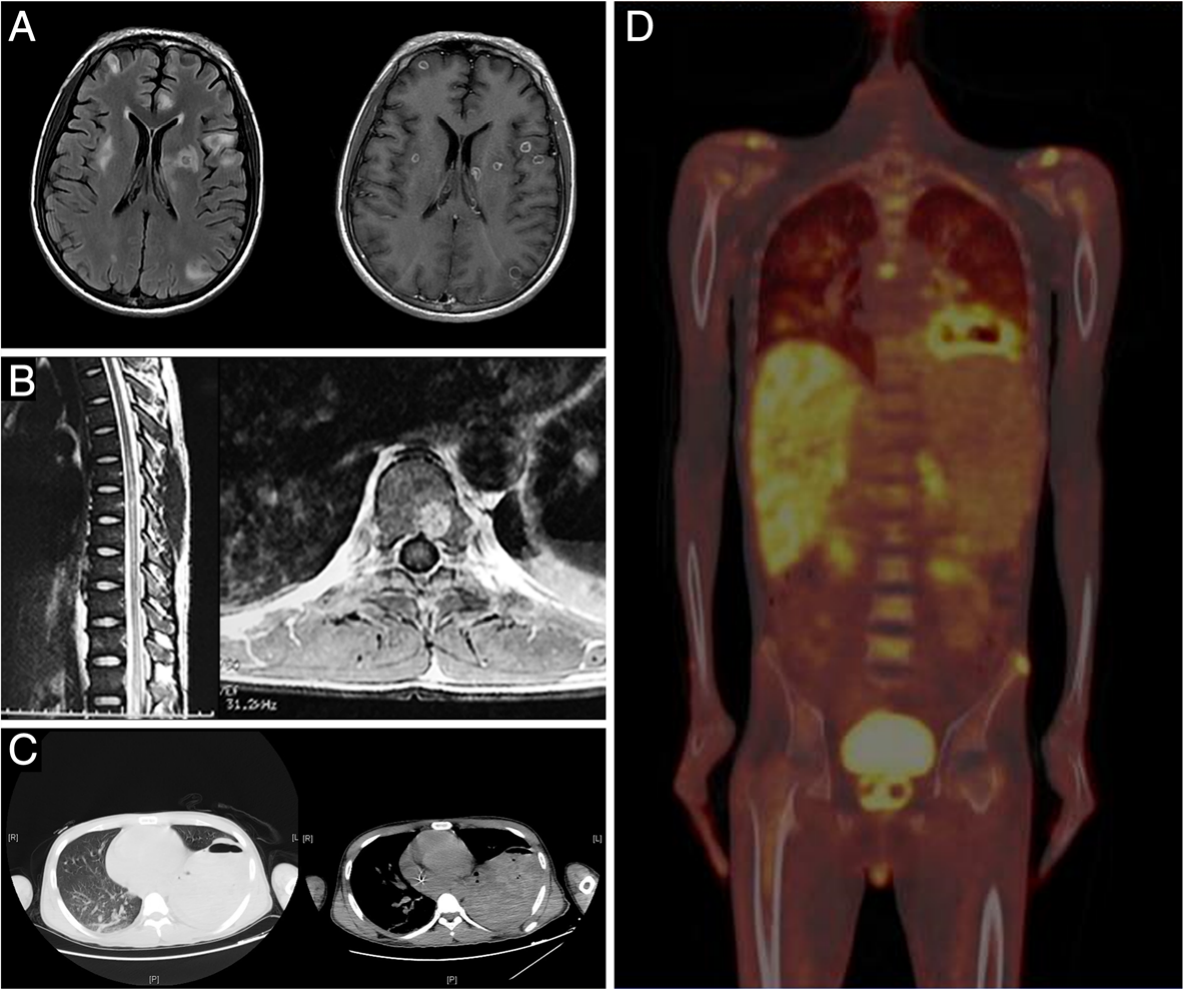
Imagine examination of the brain, spine, vertebra and lung: (a, b) Contrasted MRI were conducted on May 24th, 2017, and an axial T2-weighted image of the head found multiple lesions in the brain and abnormal signals were observed in spinal cord and vertebra in both sagittal and axial imaging. c Chest CT scan was conducted on May 28th and found multiple nodules in both lungs and a large lesion in lower left lung combined with cavity formation and gas-fluid levels inside. d PET/CT scan was conducted on June 10th and revealed abnormal uptake in the lesions of lung (SUV_max_ = 5.4), mediastinal lymph nodes (SUV_max_ = 4.4), liver (SUV_max_ = 3.8), left adrenal gland (SUV_max_ = 2.0), prostate (SUV_max_ = 6.6) and bones (SUV_max_ = 9.4). No abnormal uptake was found in brain
